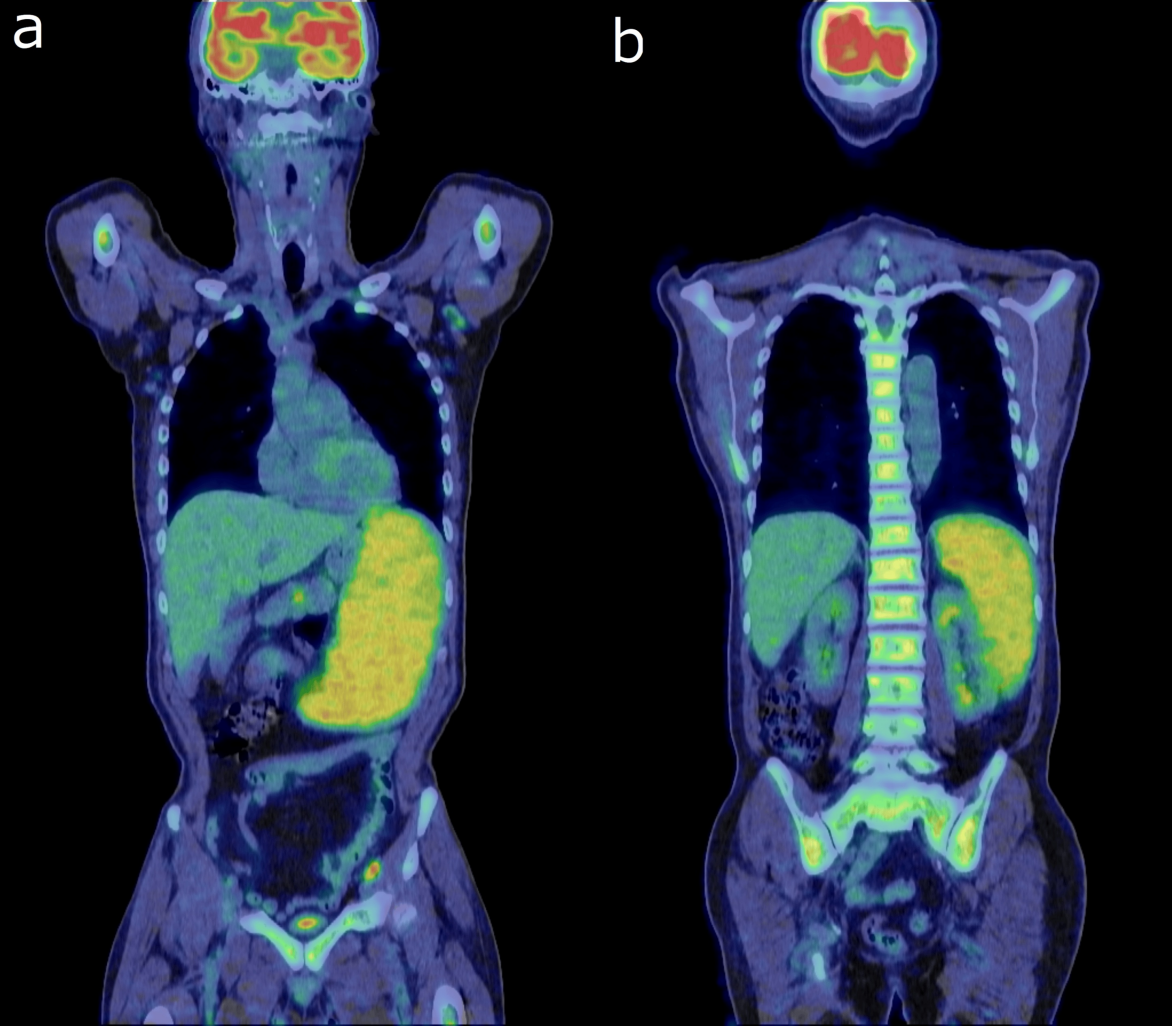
18F-FDG PET-CT 18F-fluorodeoxyglucose positron emission tomography-computed tomography (18F-FDG PET-CT) revealed intense fluorodeoxyglucose (FDG) uptake confined to the spleen (a) In addition, diffuse skeletal uptake was observed, suggestive of secondary bone marrow hyperactivity (b).
